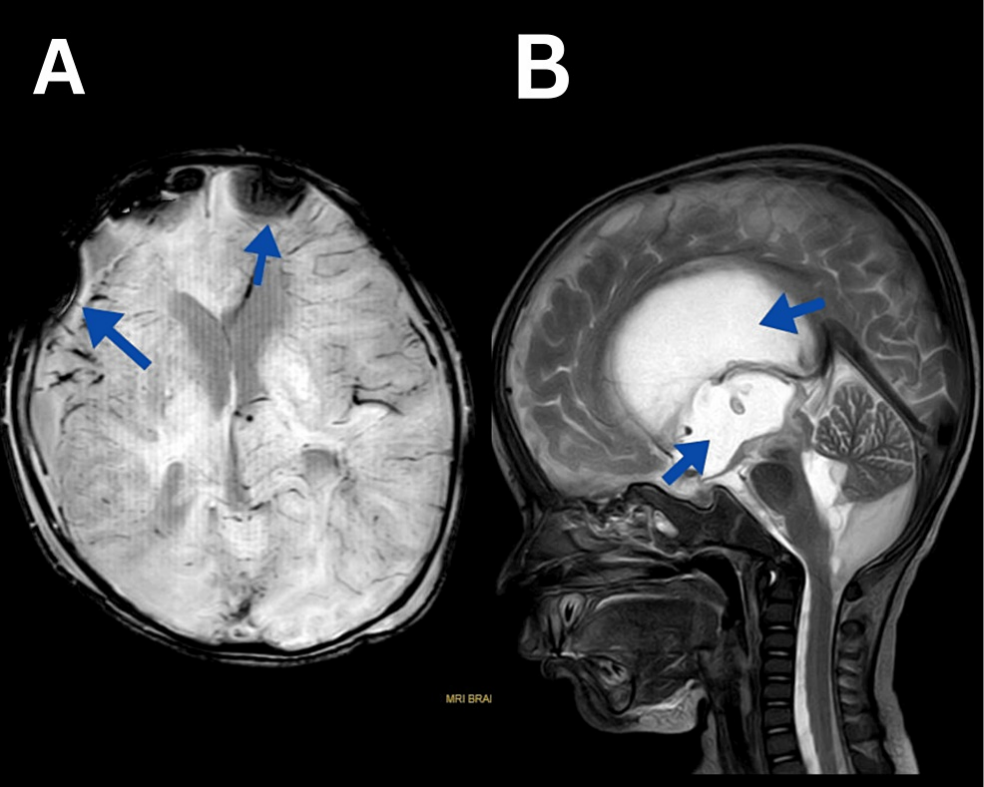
MRI findings of the brain (A) Subdural hemorrhage in the right frontal-temporal parietal and left frontoparietal region. (B) Hydrocephalus in the lateral, third ventricle, and fourth ventricle
